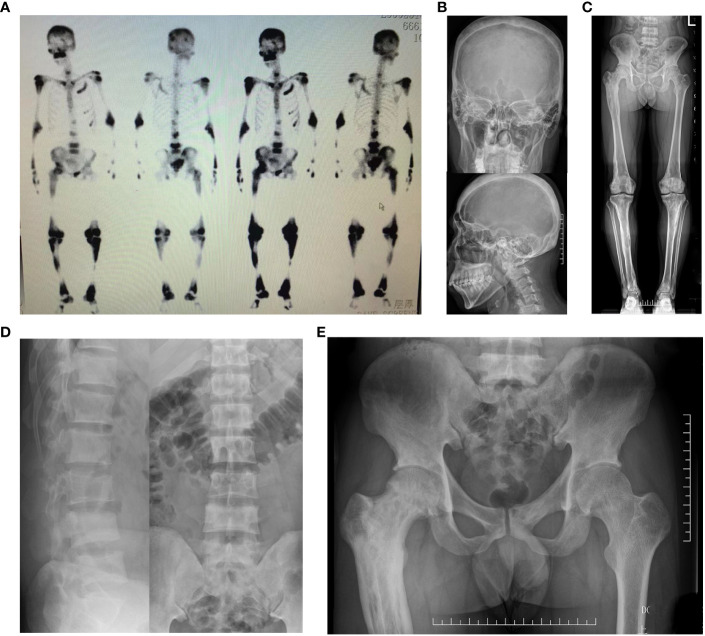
Whole-body bone scintigraphy and radiographic findings of the patient with *HNRNPA2B1* mutation. (A) Whole-body bone scintigraphy of the patient showed radionuclide uptake in multiple bones. (B) The X-ray of skull showed the thickened skull plate barrier, and the slight enlarged skull bone with diffuse sclerotic foci. (C) The radiograph of the bilateral lower extremities revealed the heterogeneous density in both lower limbs with patchy areas of osteosclerotic, and irregular destruction of the cortical bone. (D) The lumbar spine radiographs showed the increased density in vertebral bodies with slight vertebral compression. (E) The pelvic radiograph showed the increased sclerosis sites in pelvis.
